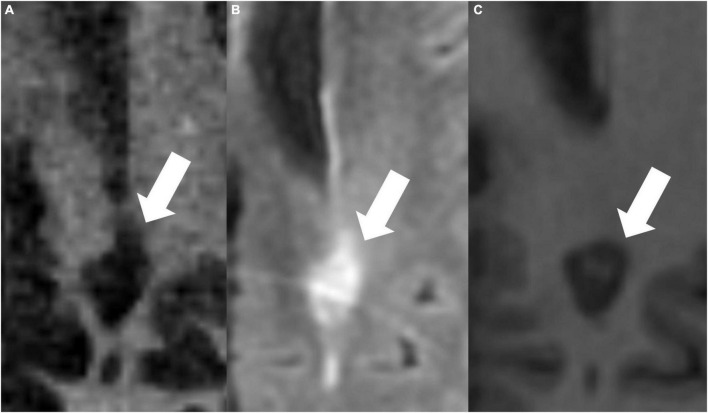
The same periventricular MS lesion (white arrows) is shown on IR-UTE (A), FLAIR (B), and MPRAGE (C) sequences, respectively.
